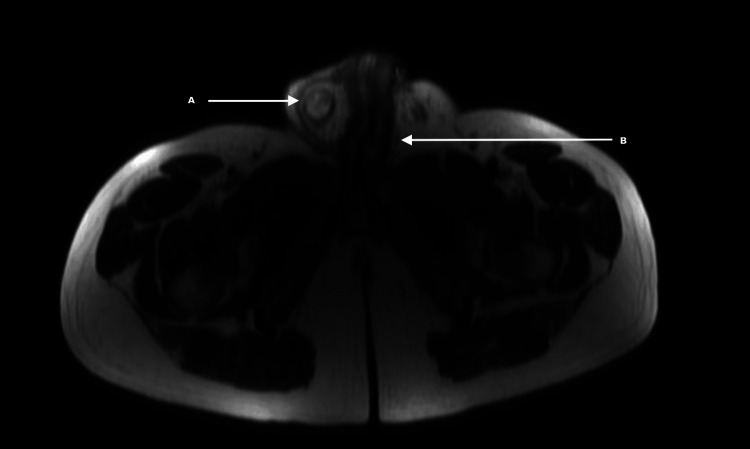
Pelvic magnetic resonance imaging demonstrating postoperative changes at the penile base and right testicular infarction Axial pelvic magnetic resonance imaging demonstrating postoperative changes involving the corpora cavernosa and corpus spongiosum at the proximal penile shaft, penile base, and intracavernosal septum, as well as right testicular infarction. A: right testis with imaging findings consistent with infarction. B: postoperative changes at the penile base
